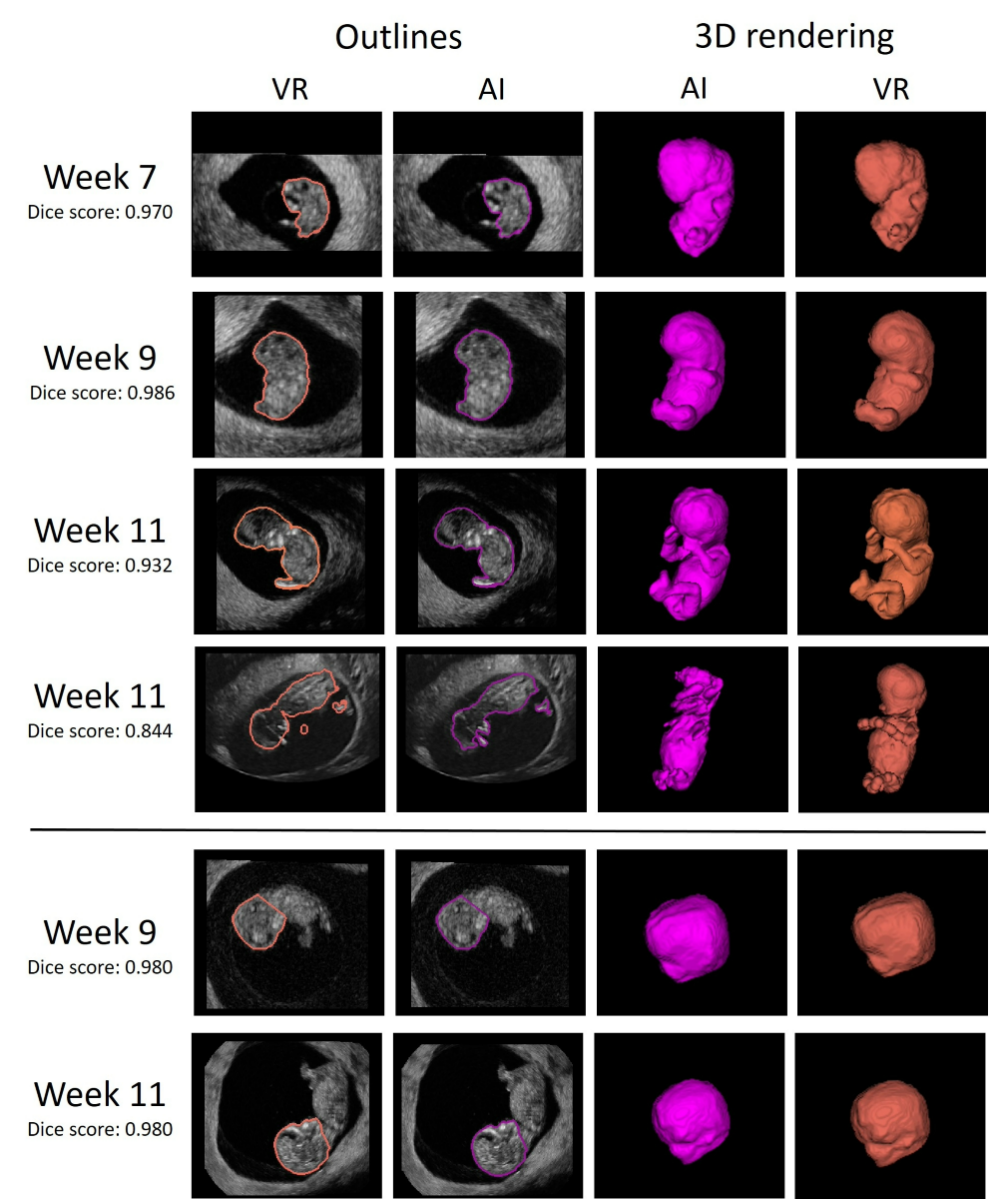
Visualization of the segmentations obtained using artificial intelligence (AI) and virtual reality (VR). For both the AI and VR segmentations on the left the outline is given, and on the right the 3D rending. Rows 1-3 show successful examples for embryonic volume (EV) measurement and column 4 shows an example where the quality of the ultrasound hampered the AI segmentation, caused by shadow near the fetal head. Rows 5 and 6 show successful examples of head volume measurement.
